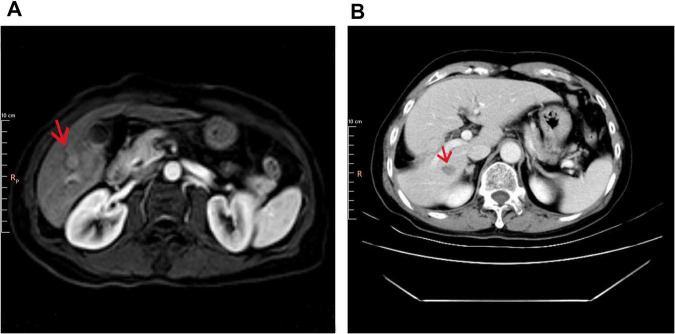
Imaging findings at baseline and post-operation. (A) Baseline liver imaging with abdominal MRI (T1 post-contrast, arterial phase). The arrow indicates mild liver cirrhosis and a 2 cm hypervascular mass in the right hepatic lobe (Segment VI). (B) Postoperative CT (Portal Venous Phase) showing surgical resection of liver segments V and VI.
